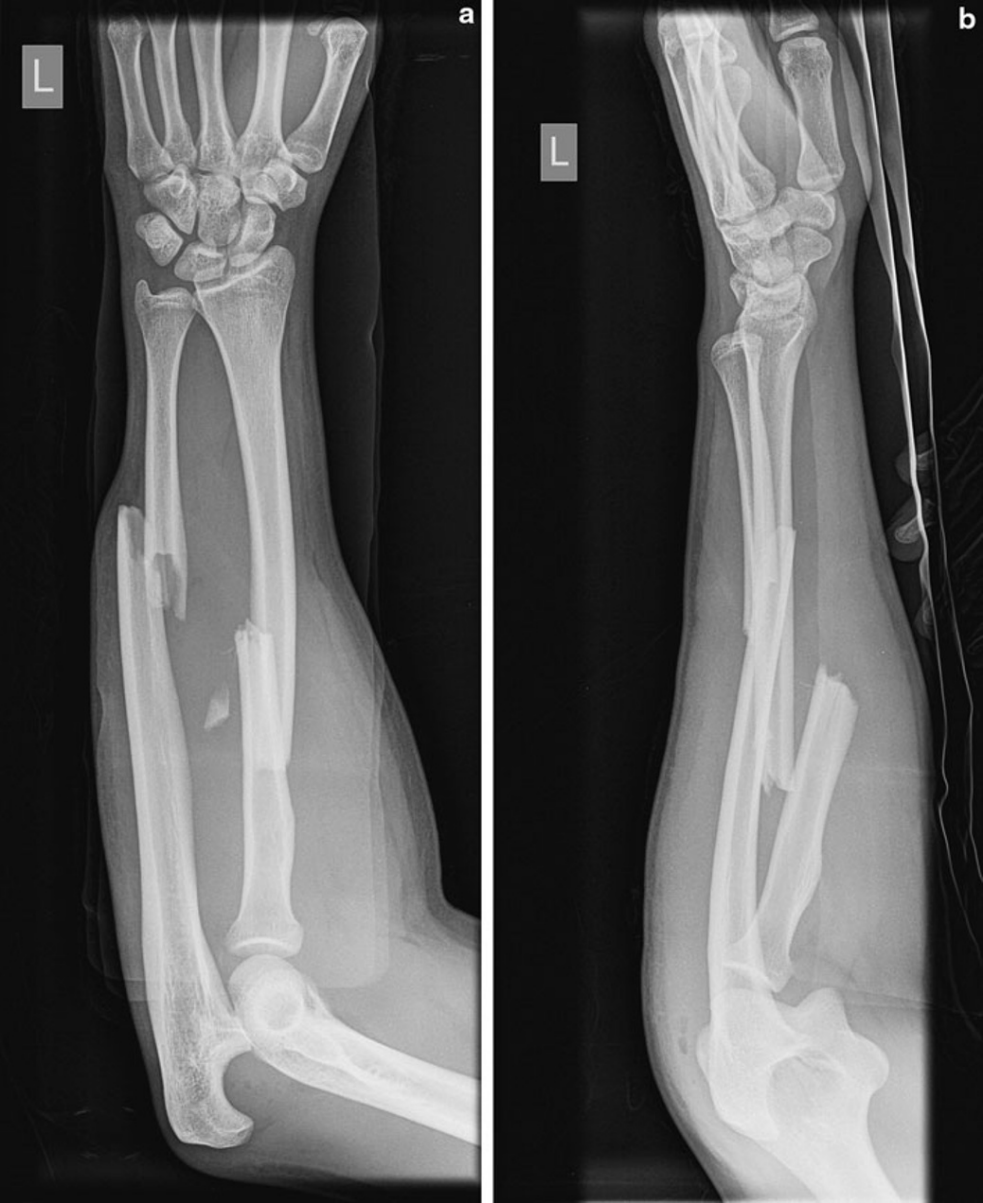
a, b Preoperative anteroposterior and lateral radiographs showing radius and ulna fractures with isolated ulno-humeral posterior dislocation
